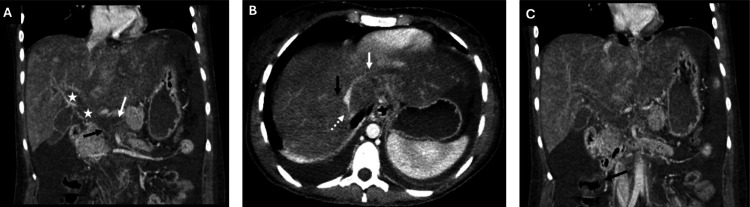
Pre-procedure abdominal CT. (A) Thrombosis of the intrahepatic and extrahepatic portal veins (white star), splenic vein (white arrow), and superior mesenteric vein (black arrow). (B) Thrombosis of the right (dotted white arrow), middle (solid black arrow), and left (solid white arrow) hepatic veins. (C) Bowel wall thickening of the proximal ascending colon (black arrow).
